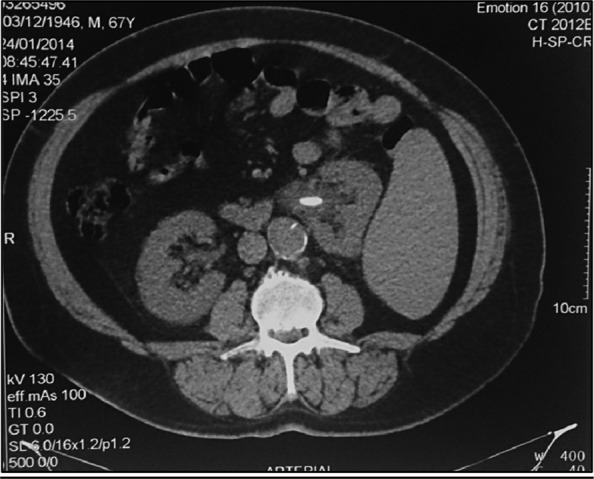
Abdominal CT that evidences splenomegaly and nephrolithiasis.
